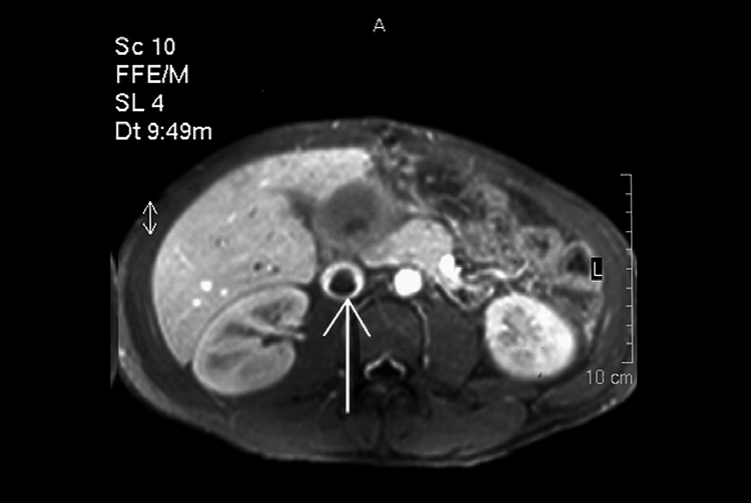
MRI revealing a thrombus in the inferior vena cava (the arrow shows the thrombus)].
